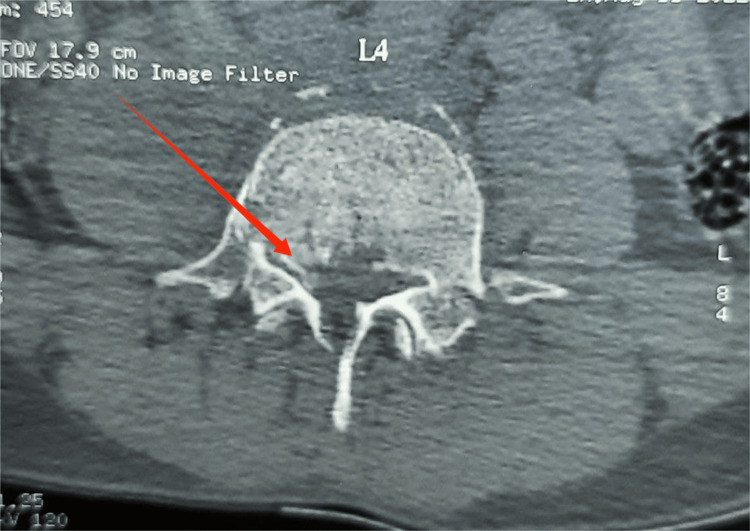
CT showing L4 burst fracture with retropulsion of fragment
